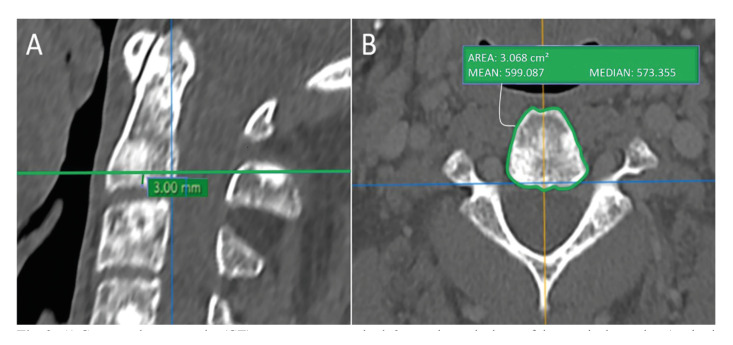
A) Computed tomography (CT) measurement method, 3 mm above the base of the cervical vertebra (sagittal image). B) Region of interest (ROI) was set on the C2 body region contour including medullary and cortical bones - mean and mean CT grayscale values (axial image).
